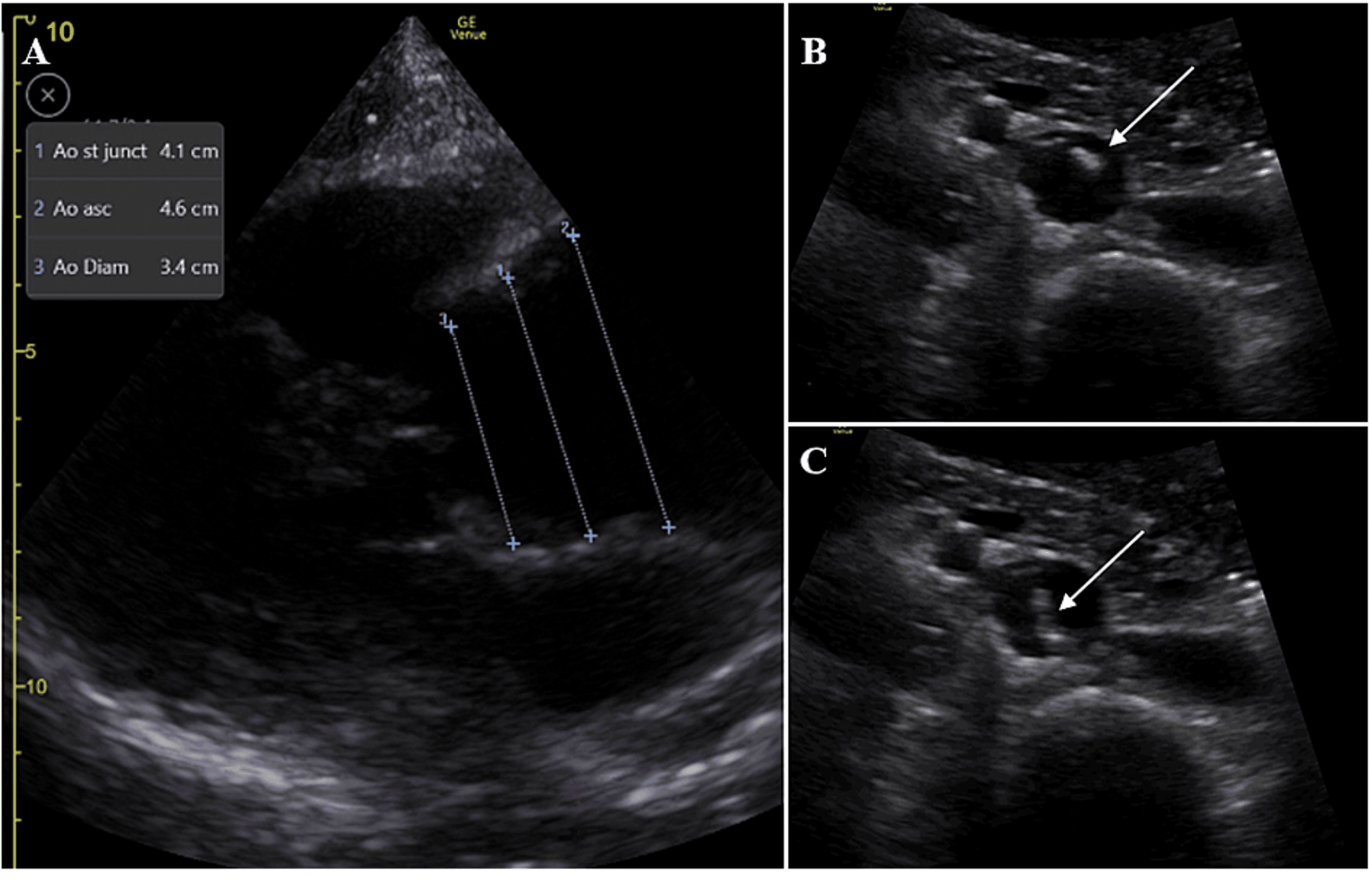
PoCUS showing a dilated aortic root in the parasternal long-axis view (A) and a mobile flap (white arrow) in the abdominal aorta in the transverse view (B, C) PoCUS: point-of-care ultrasound
